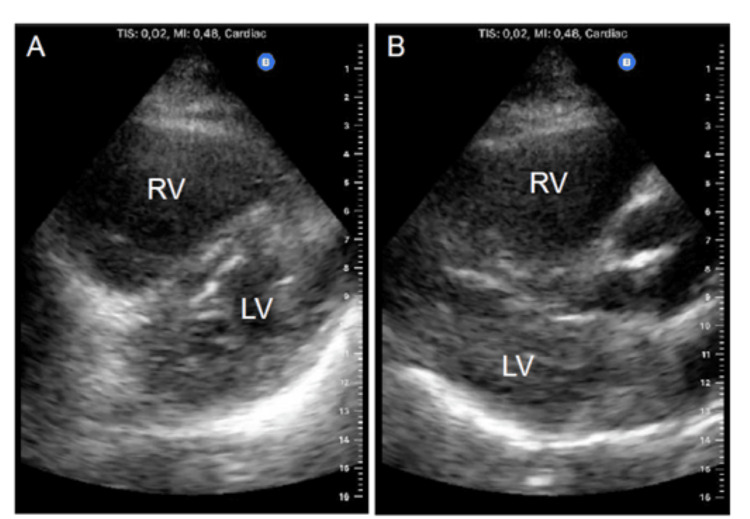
POCUS in cardiac arrest. (A) Parasternal short axis; (B) parasternal long axis. Images were obtained using a Butterfly iQ™ probe. POCUS: point-of-care ultrasound; RV: right ventricle; LV: left ventricle.
